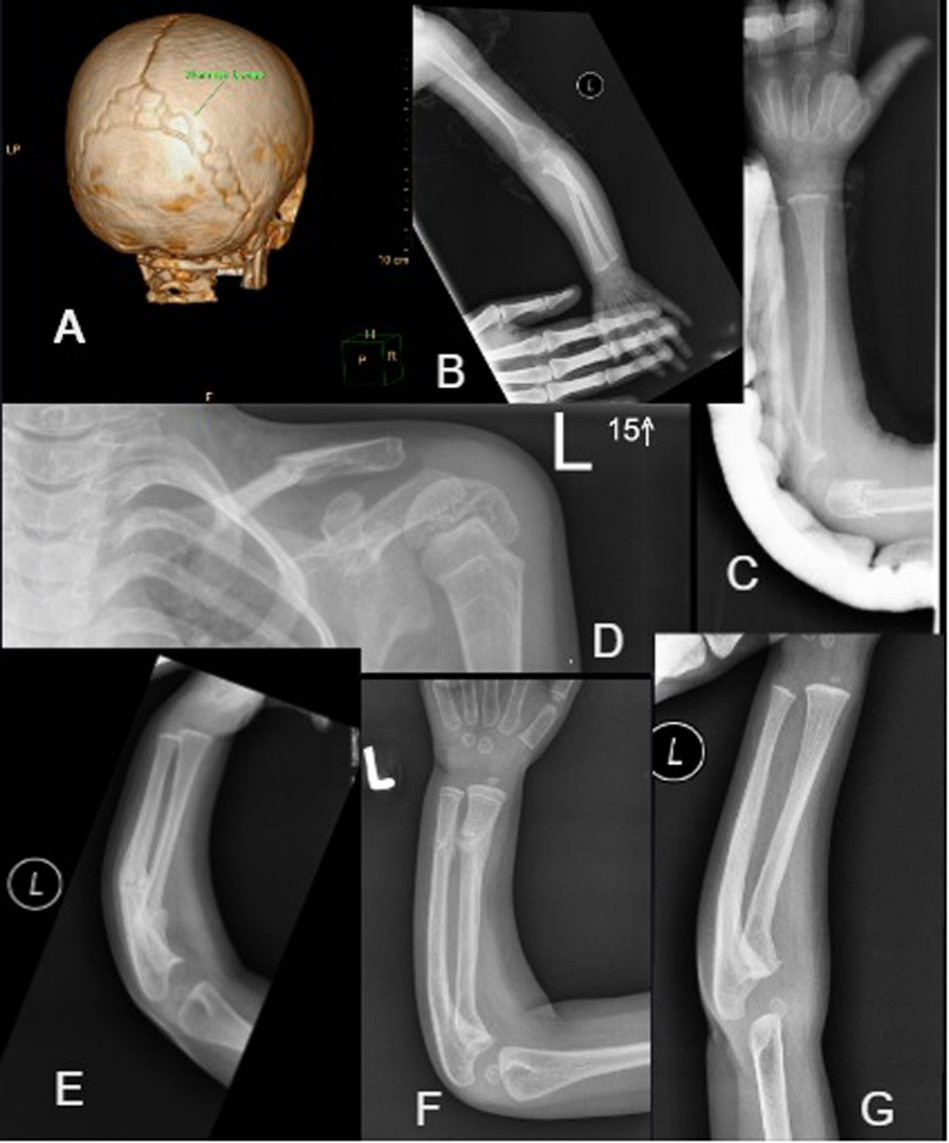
Radiographs of patient PXYU (*IFITM5*). A CT skull (age 2.5 years): showing numerous Wormian bones. B Fracture left ulna (midshaft) at 13 months—note no obvious callous formation. C Fracture distal ulna and radius at 23 months. D Fracture left clavicle at age 4 years (note zebra stripes on proximal humerus from bisphosphonate therapy). E Fracture midshaft ulna and radius at 14 months with mild callus formation. F & G Healed proximal ulna/radius fracture at 19 months.
